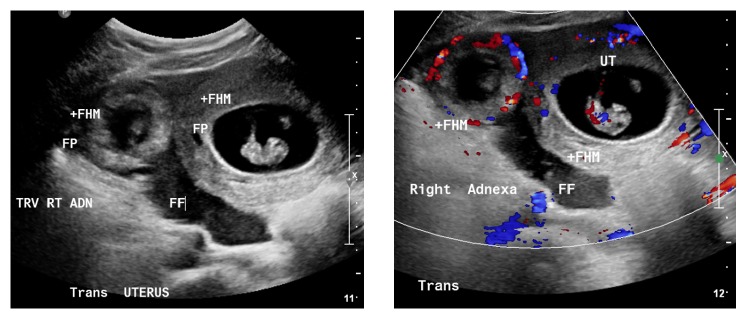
Patient number 1. Pelvic ultrasound showing the intrauterine and extrauterine/tubal ectopic pregnancies, both with fetal pole (FP), fetal heart rates (+FHM) present, and free fluid (FF) in the peritoneal cavity.
